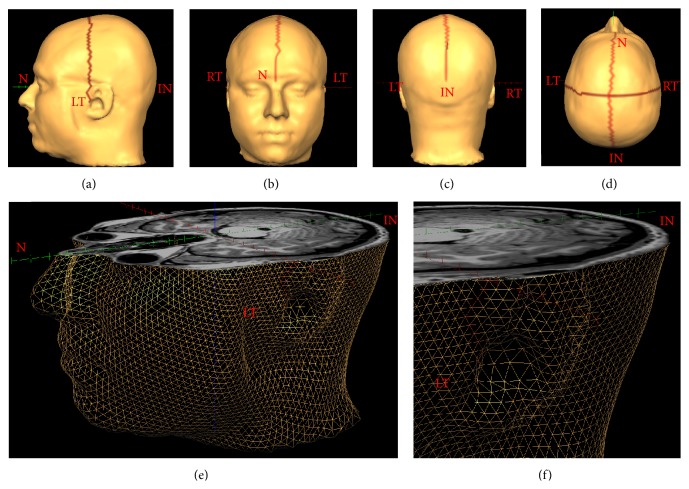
A 3D mesh morphed to the structural MRI data of a representative participant. Panels (a)–(d) show the anatomical landmarks that were used as anchor points for scalp distance calculations marked on a T1-volume surface mesh created using Brain Voyager. N: nasion, RT and LT: right and left tragi, respectively, and IN: inion. The lines connecting the anatomical landmarks are “patches of interest” (POIs) drawn in Brain Voyager that link adjacent triangles in the mesh. Panels (e) and (f) show close-up views of the mesh without the surface coloring. The mesh has been cut axially at the level of the inion. The smooth surface of the head is represented using triangular elements and each of these elements is defined by its tricorners.
